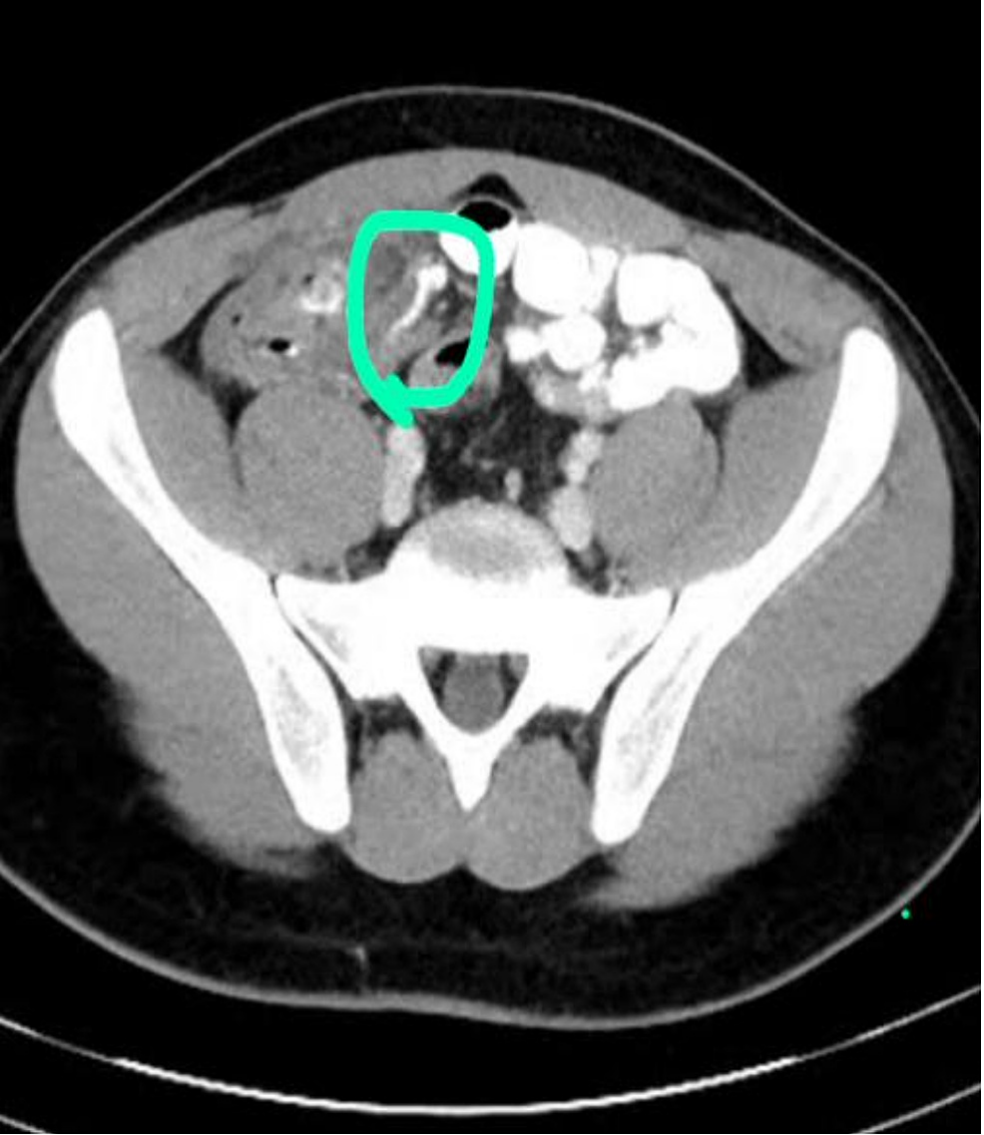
CECT scan of the abdomen-pelvis showing the appendix to be significantly thickened with peri-appendiceal fat stranding. CECT, contrast-enhanced computed tomography
